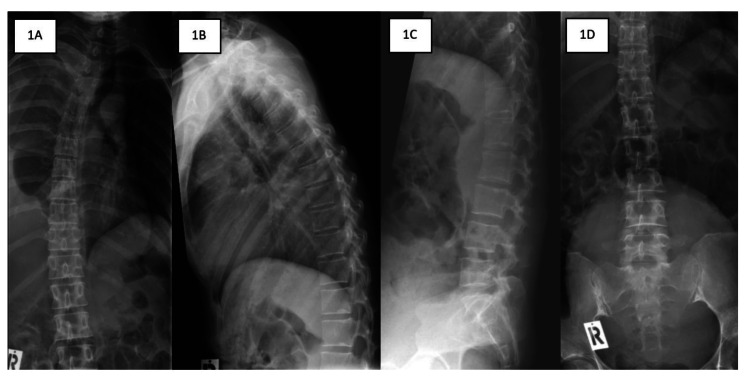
The plain radiographs of the thoracic and lumbar spine revealed no gross abnormality.
